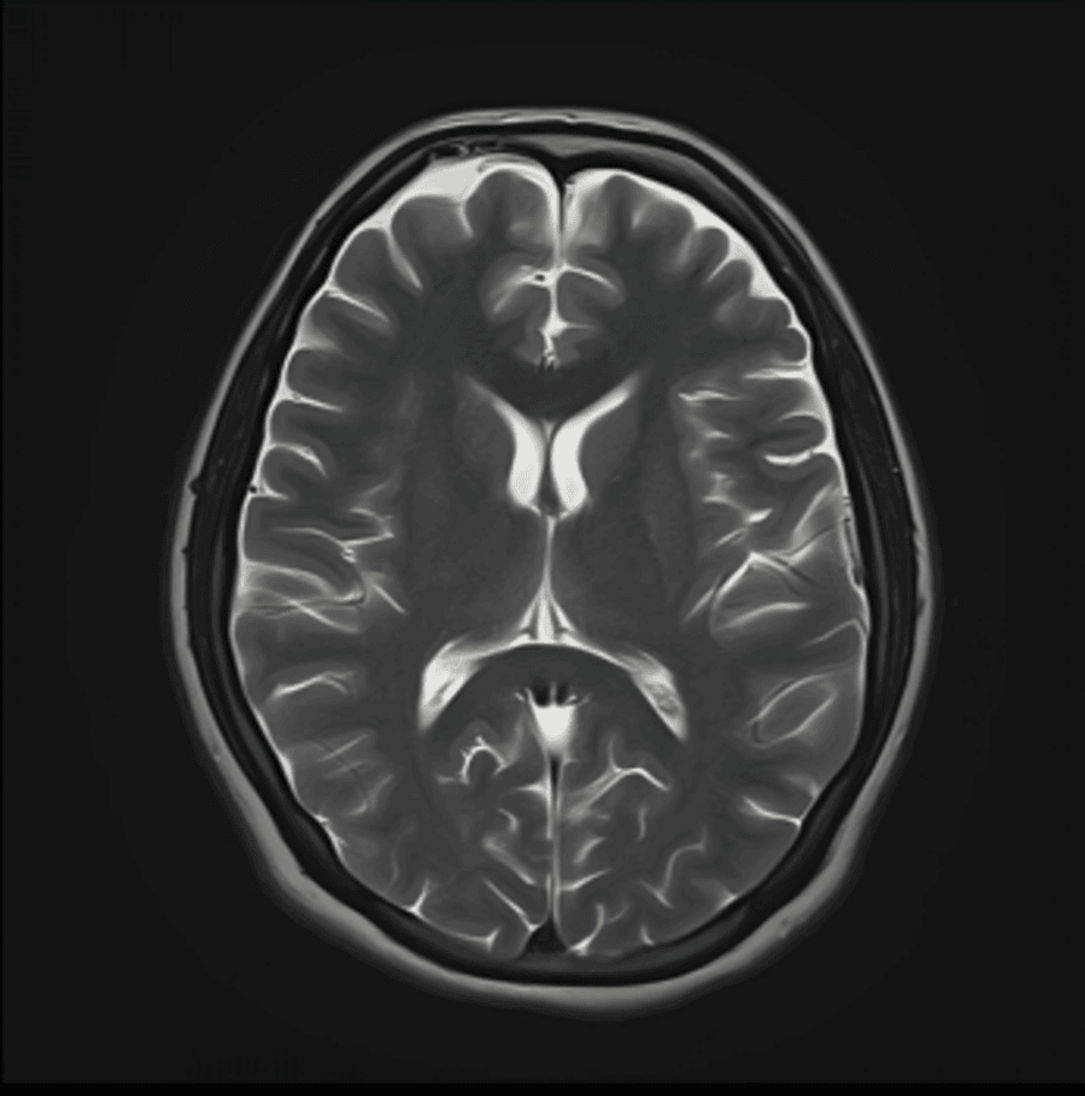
Normal axial T2-weighted magnetic resonance image (MRI) of the brain without contrast
